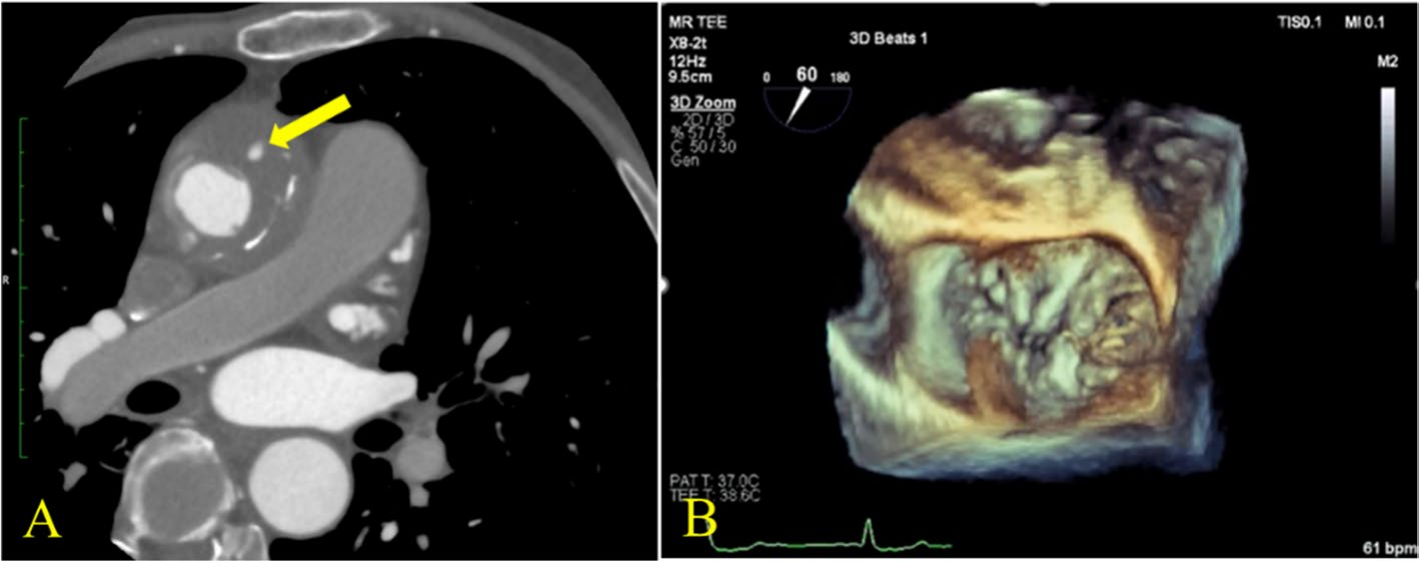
A Preoperative contrast-enhanced CT showing contrast leakage at the distal anastomosis following Bentall procedure. B Preoperative transesophageal echocardiography demonstrating extensive degenerative change of both leaflets and P3 prolapse due to chordal rupture
